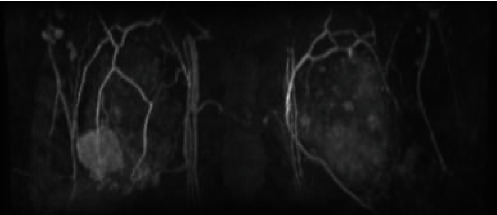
Magnetic resonance imaging revealed a fast plateau pattern with a mass measuring 35 mm in the right breast and bilateral lymphadenopathy.
